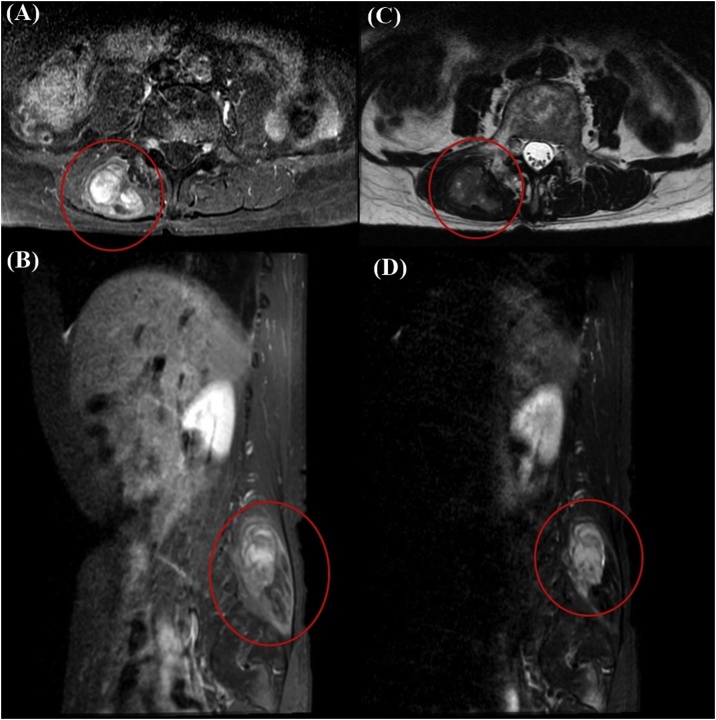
MRI image of the tumor. Horizontal section (A). Sagittal section (B) of T1-contrast WIs. Horizontal section of T2 WIs (C). Sagittal section of fat suppression T2 WIs (D).
